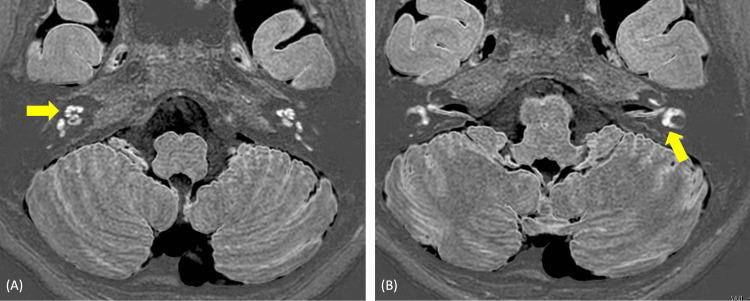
Cochlear and vestibular MRI. A: Yellow arrow indicates cochlea. B: Yellow arrow indicates vestibular. HYDROPS showed enhanced contrast effects on bilateral cochlear and vestibular.
